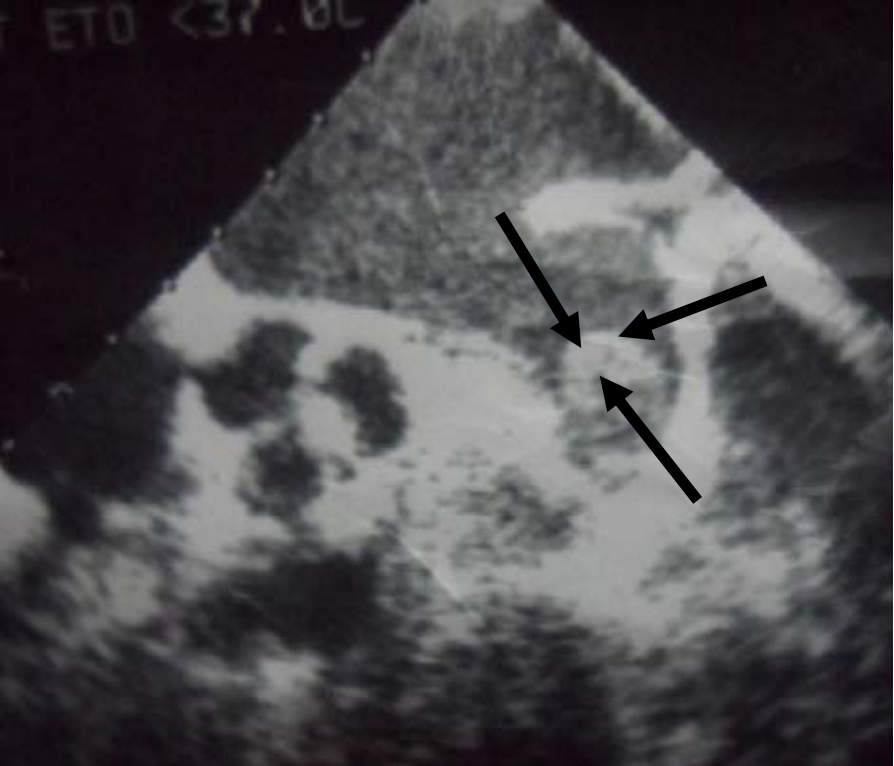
Transesophageal echocardiography: presence of a thrombus in the left atrium.
